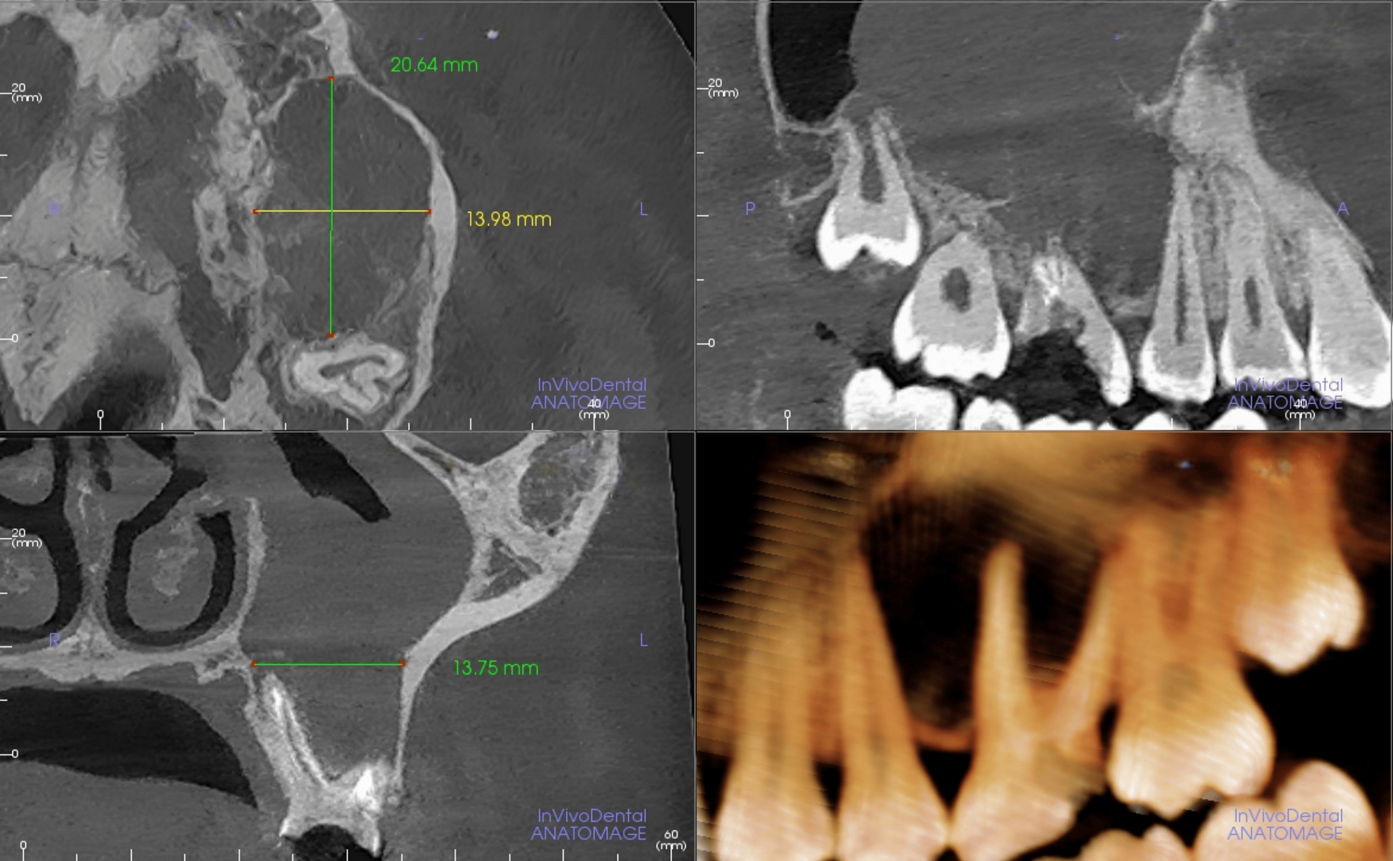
Multiplanar reconstruction of CBCT showing a hypodense lesion in the left maxillary region
